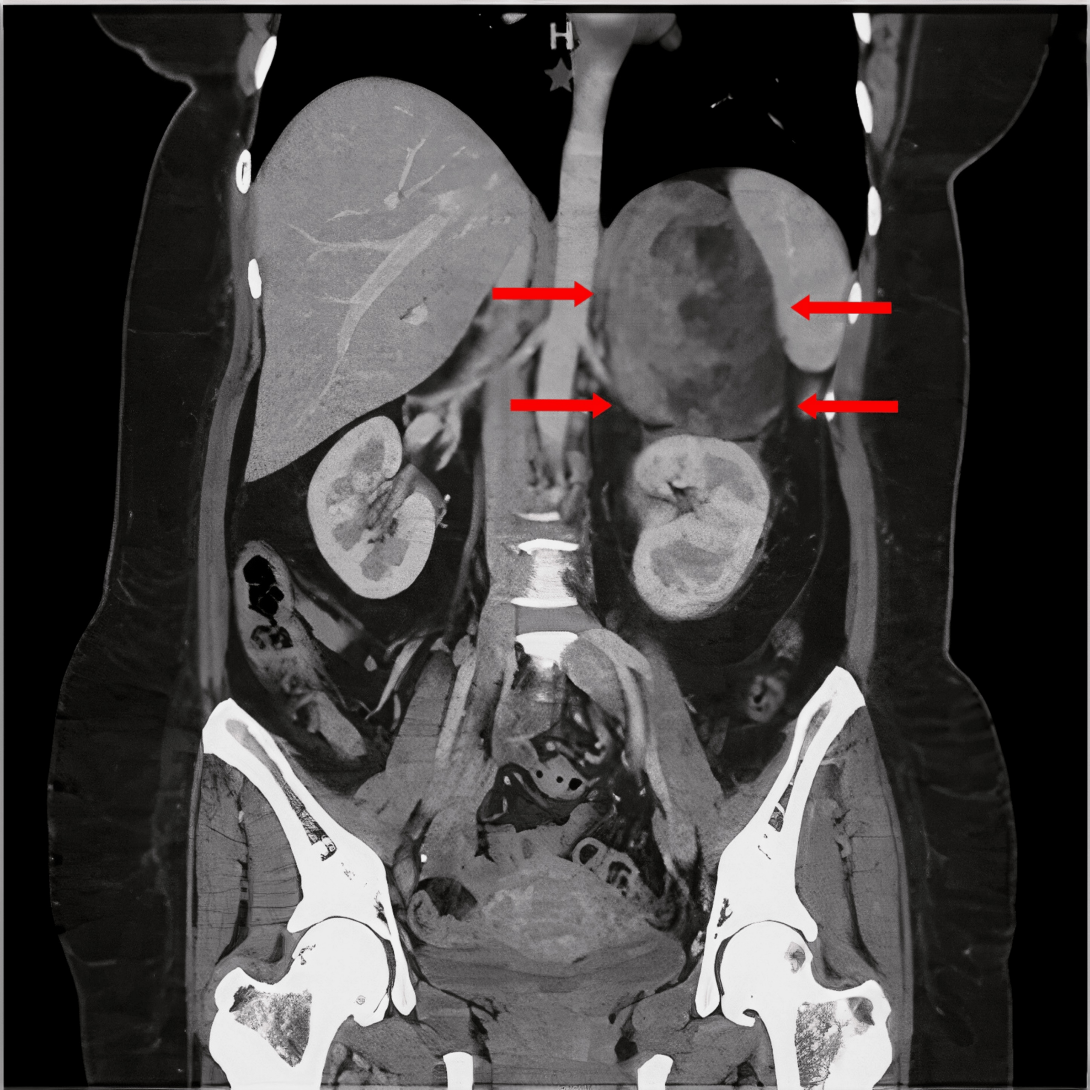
Computed tomography coronal section showing adrenal location mass with the dimensions of 90 x 69 x 62 mm that has contact with the spleen.
